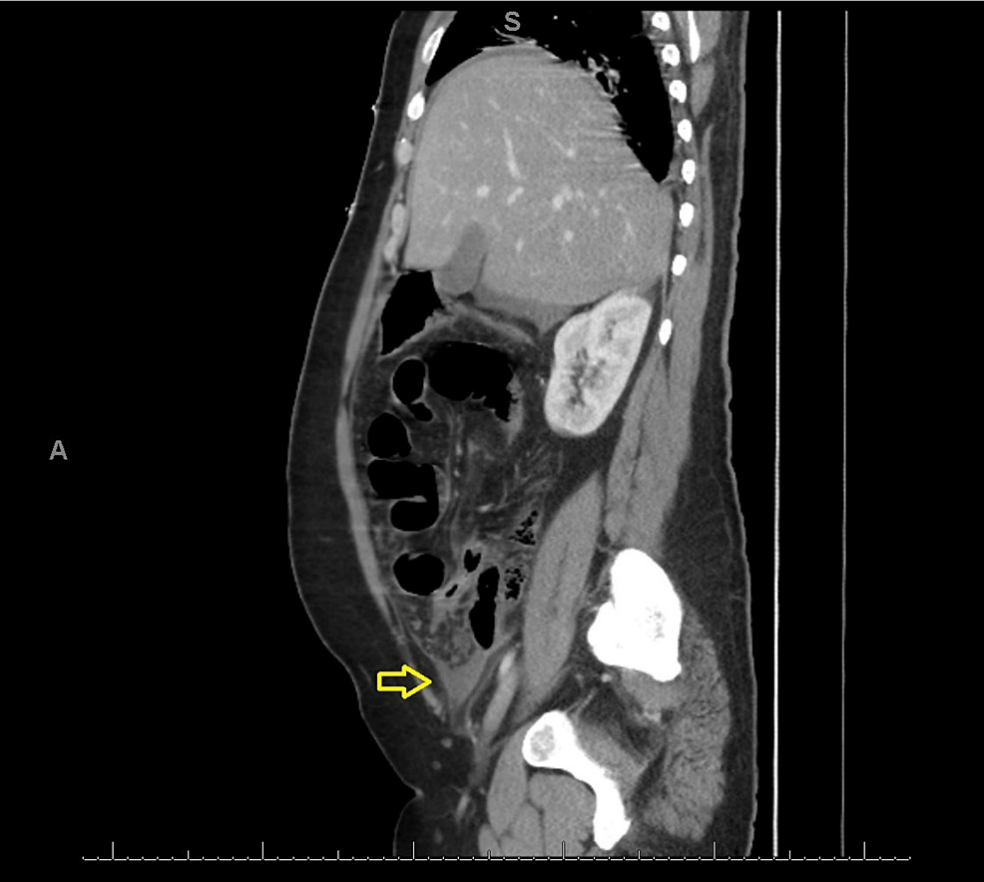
A sagittal view of an abdominal CT scan shows findings of perforated appendicitis. The area of pus collection is labeled with an arrow, and there is no extension of pus collection into the right inguinal canal.
